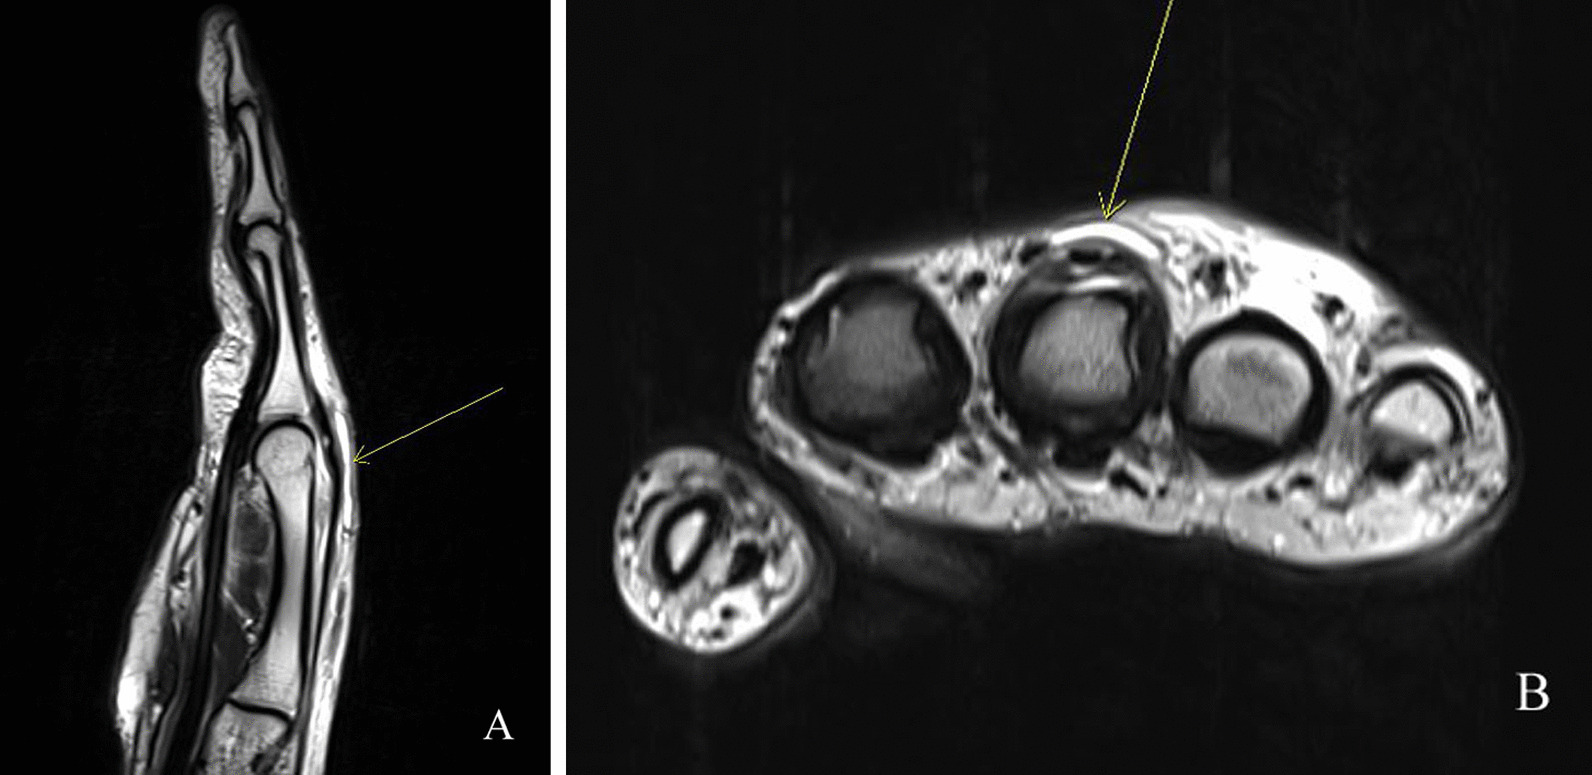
Preoperative enhanced magnetic resonance imaging of the hand. A thin elongated fluid collection at the dorsal aspect of 3rd finger MCP joint level, enhancement along the extensor tendon of 3rd finger, joint effusion with thick enhancing synovium at 3rd MCP joint suggest abscess, chronic extensor tenosynovitis, and septic arthritis, respectively, on gadolinium-enhanced T2-weighted sagittal (A) and axial (B) images. Yellow arrow in both (A) and (B) indicates 3rd MCP joint
